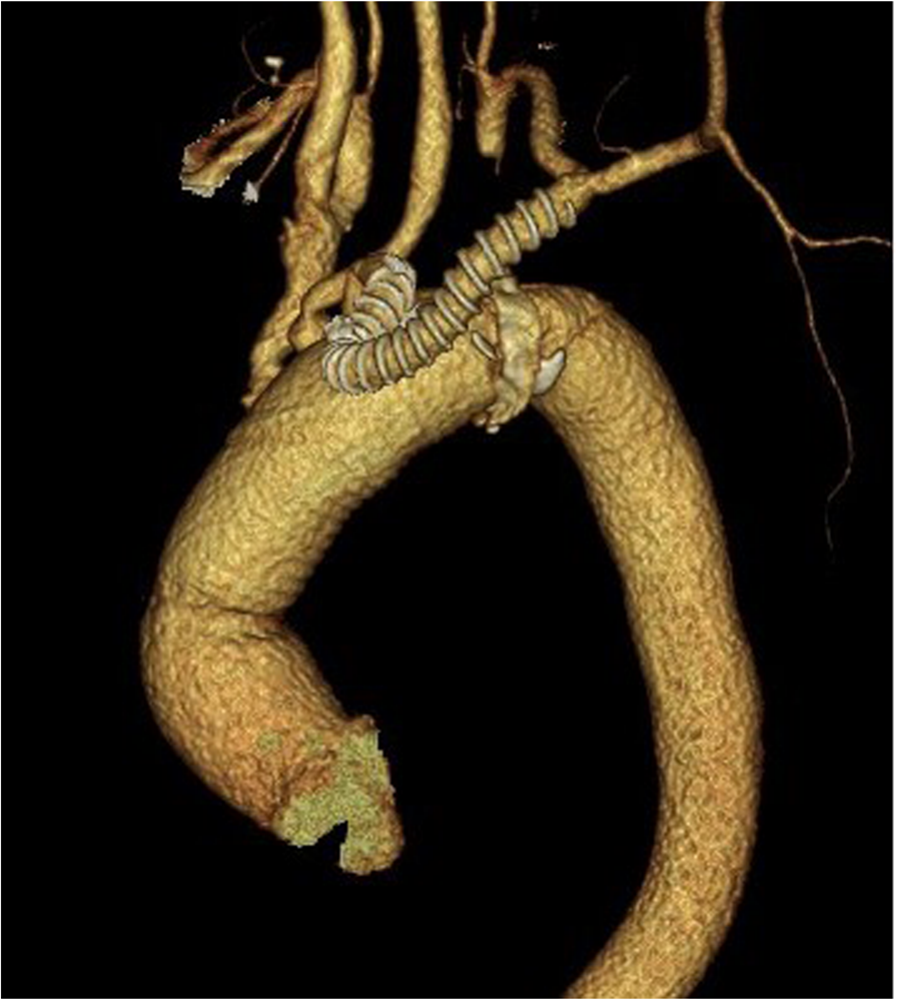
Postoperative computed tomography (CT) showed no aneurysm of the aortic arch and complete resection of the lesion
